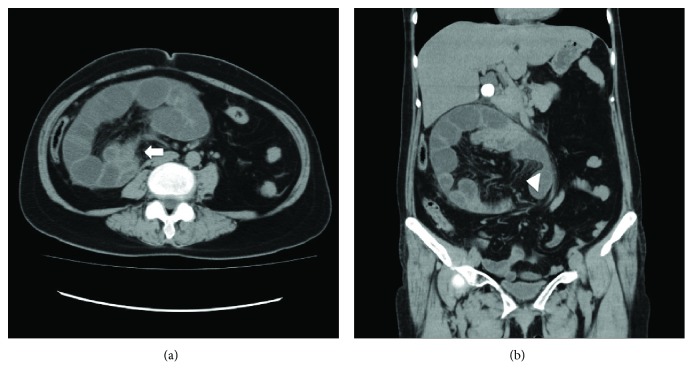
Abdominal CT (noncontrast-enhanced) findings in this patient. (a) Axial image showing that most of the small intestine was located in the right upper abdominal cavity and had a sac-like appearance (arrow). Ascites was observed within the sac. (b) Coronal image showing absence of the ligament of Treitz in the duodenum, along with a flattened inferior vena cava and distorted mesenteric veins (arrowhead). A 2 cm-sized gallstone was also observed.
